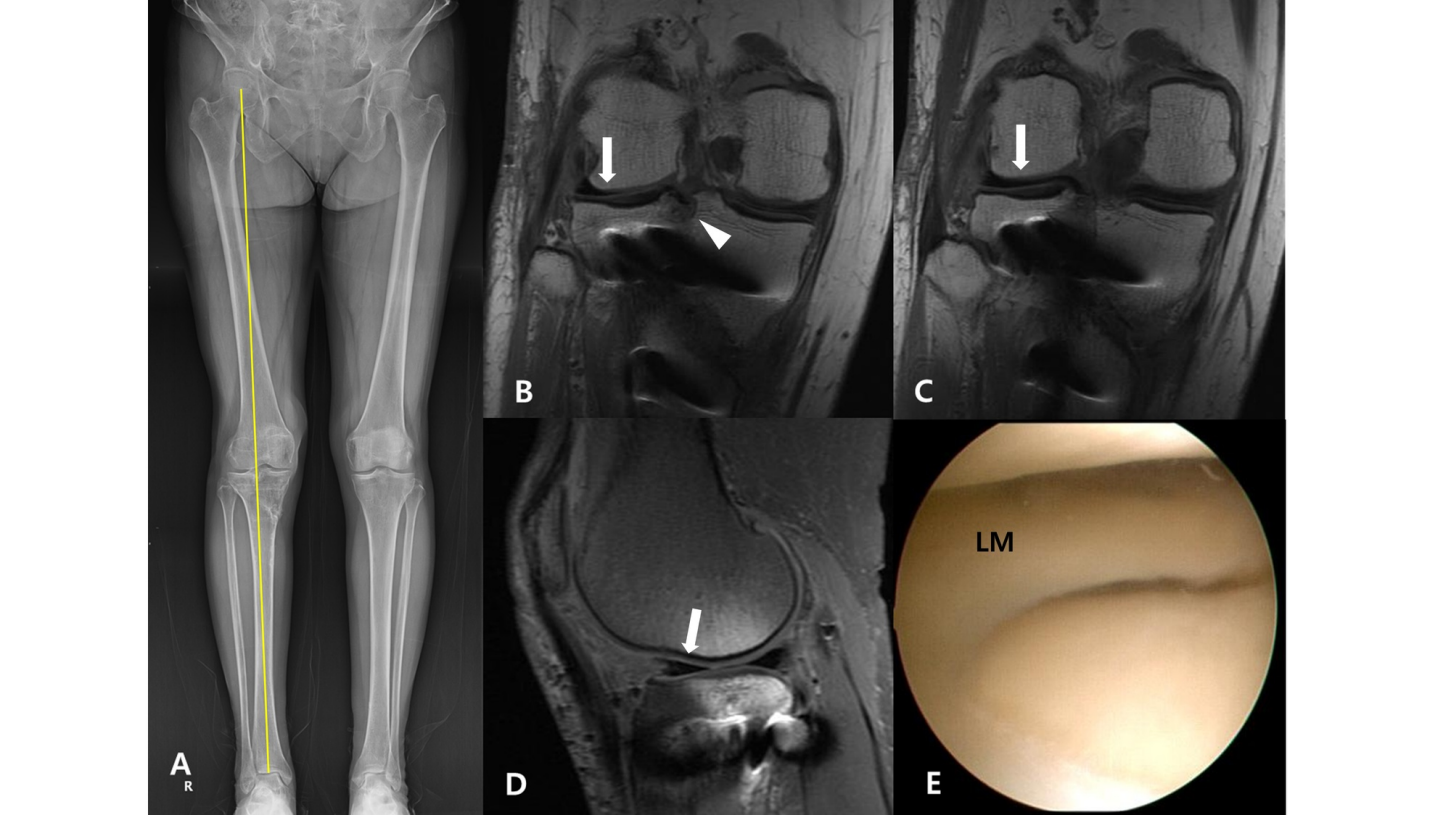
Postoperative findings. (A) Postoperative weight-bearing full-length radiogram shows union of osteotomy and correction of knee alignment to slight valgus (2.7°) angle. (B-D) Magnetic resonance imaging (MRI) taken at three months after the surgery shows intact lateral meniscus allograft (arrows) and bony bridge of the graft (arrowhead) that is unviolated by the proximal screws. (E) Second look arthroscopic examination done at postoperative one-year shows intact lateral meniscus graft.
